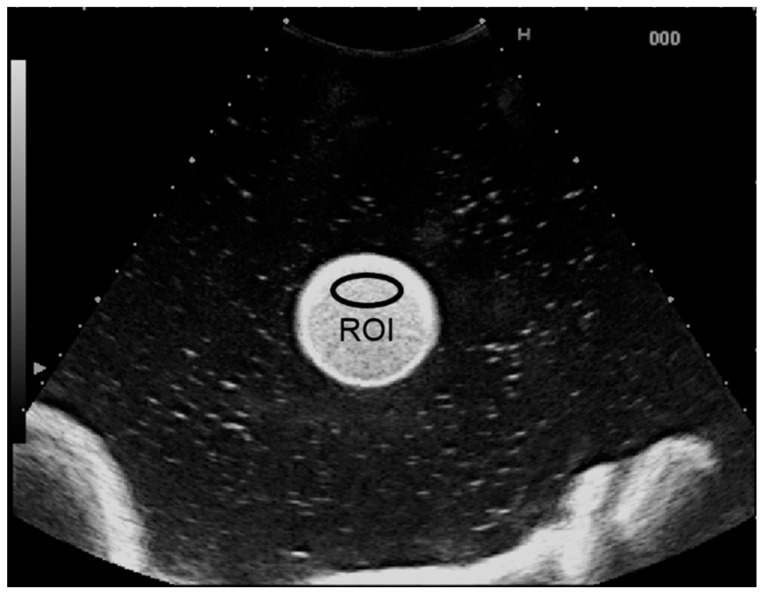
Ultrasound image of the contrast agent solution. The contrast signal intensity (CI) was measured in the region of interest (ROI) placed within the solution.
